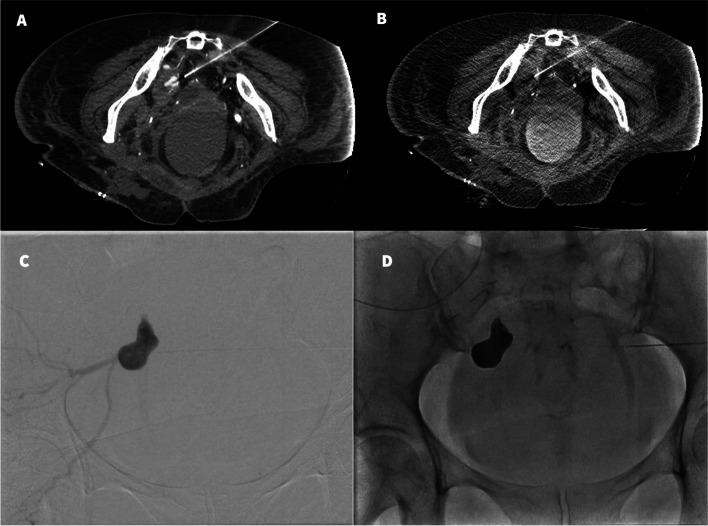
Direct percutaneous access to the mycotic pseudoaneurysm under CT guidance. A) Tip of the 20 G needle medial to the left internal iliac artery mycotic pseudoaneurysm sac on CT angiogram just before access to the sac. B) Direct access into the sac with 20 G needle. C) Contrast injection under fluoroscopy opacifies the left internal iliac artery mycotic pseudoaneurysm sac and feeding arteries. D) Onyx embolization of the mycotic pseudoaneurysm sac
